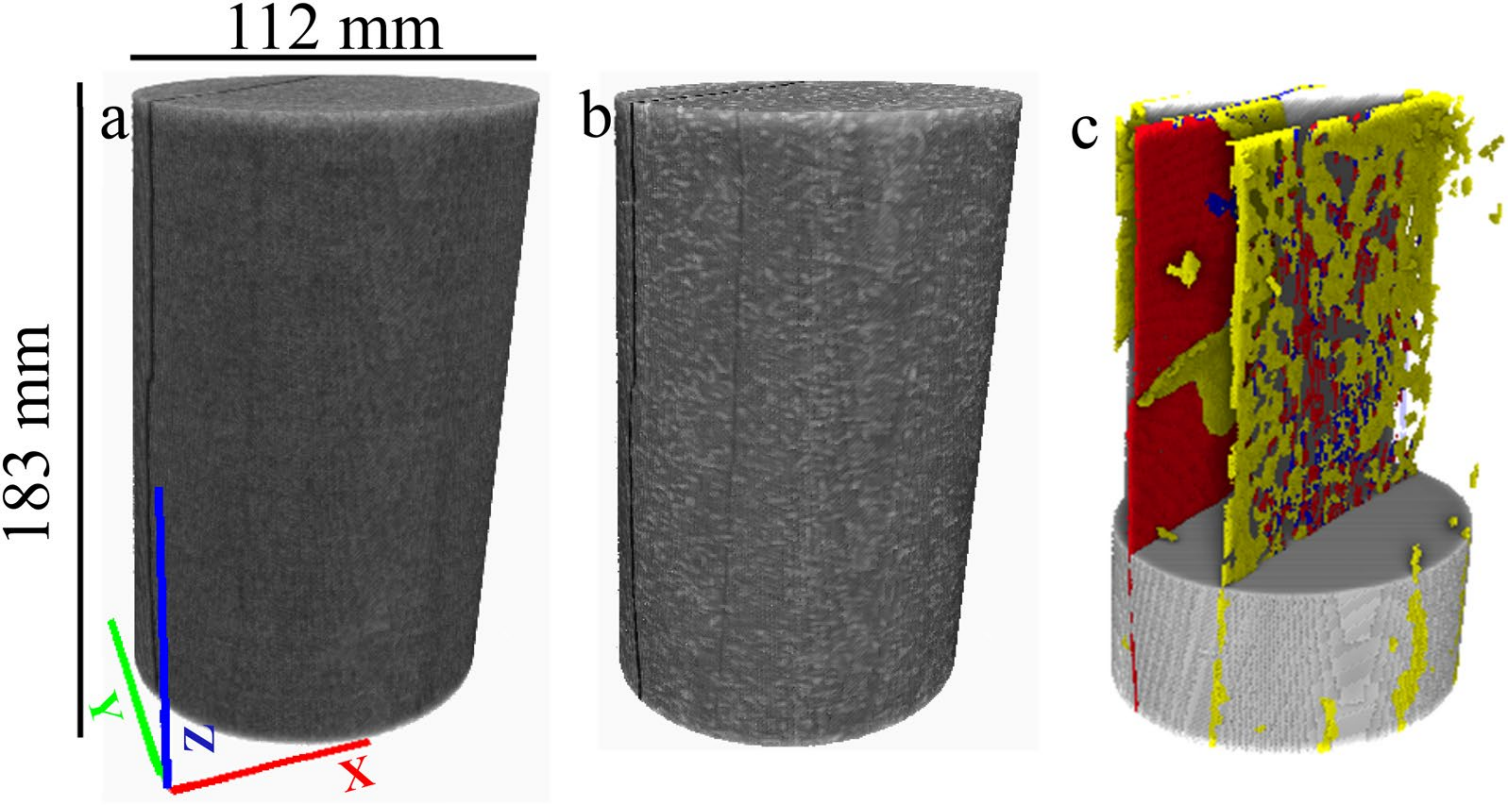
3D representations of ORS: (a) the greyscale neutron tomography image (black = fractures/micro-fractures, grey = solid matrix, (b) the grey-scale µ-CT image (black = fractures/micro-fractures, grey and white = organic and inorganic minerals), and (c) segmented image (grey = solid phase, red: empty fractures, blue = fluid-saturated fracture spaces and yellow = liquid diffused into the matrix adjacent to micro-fractures).
